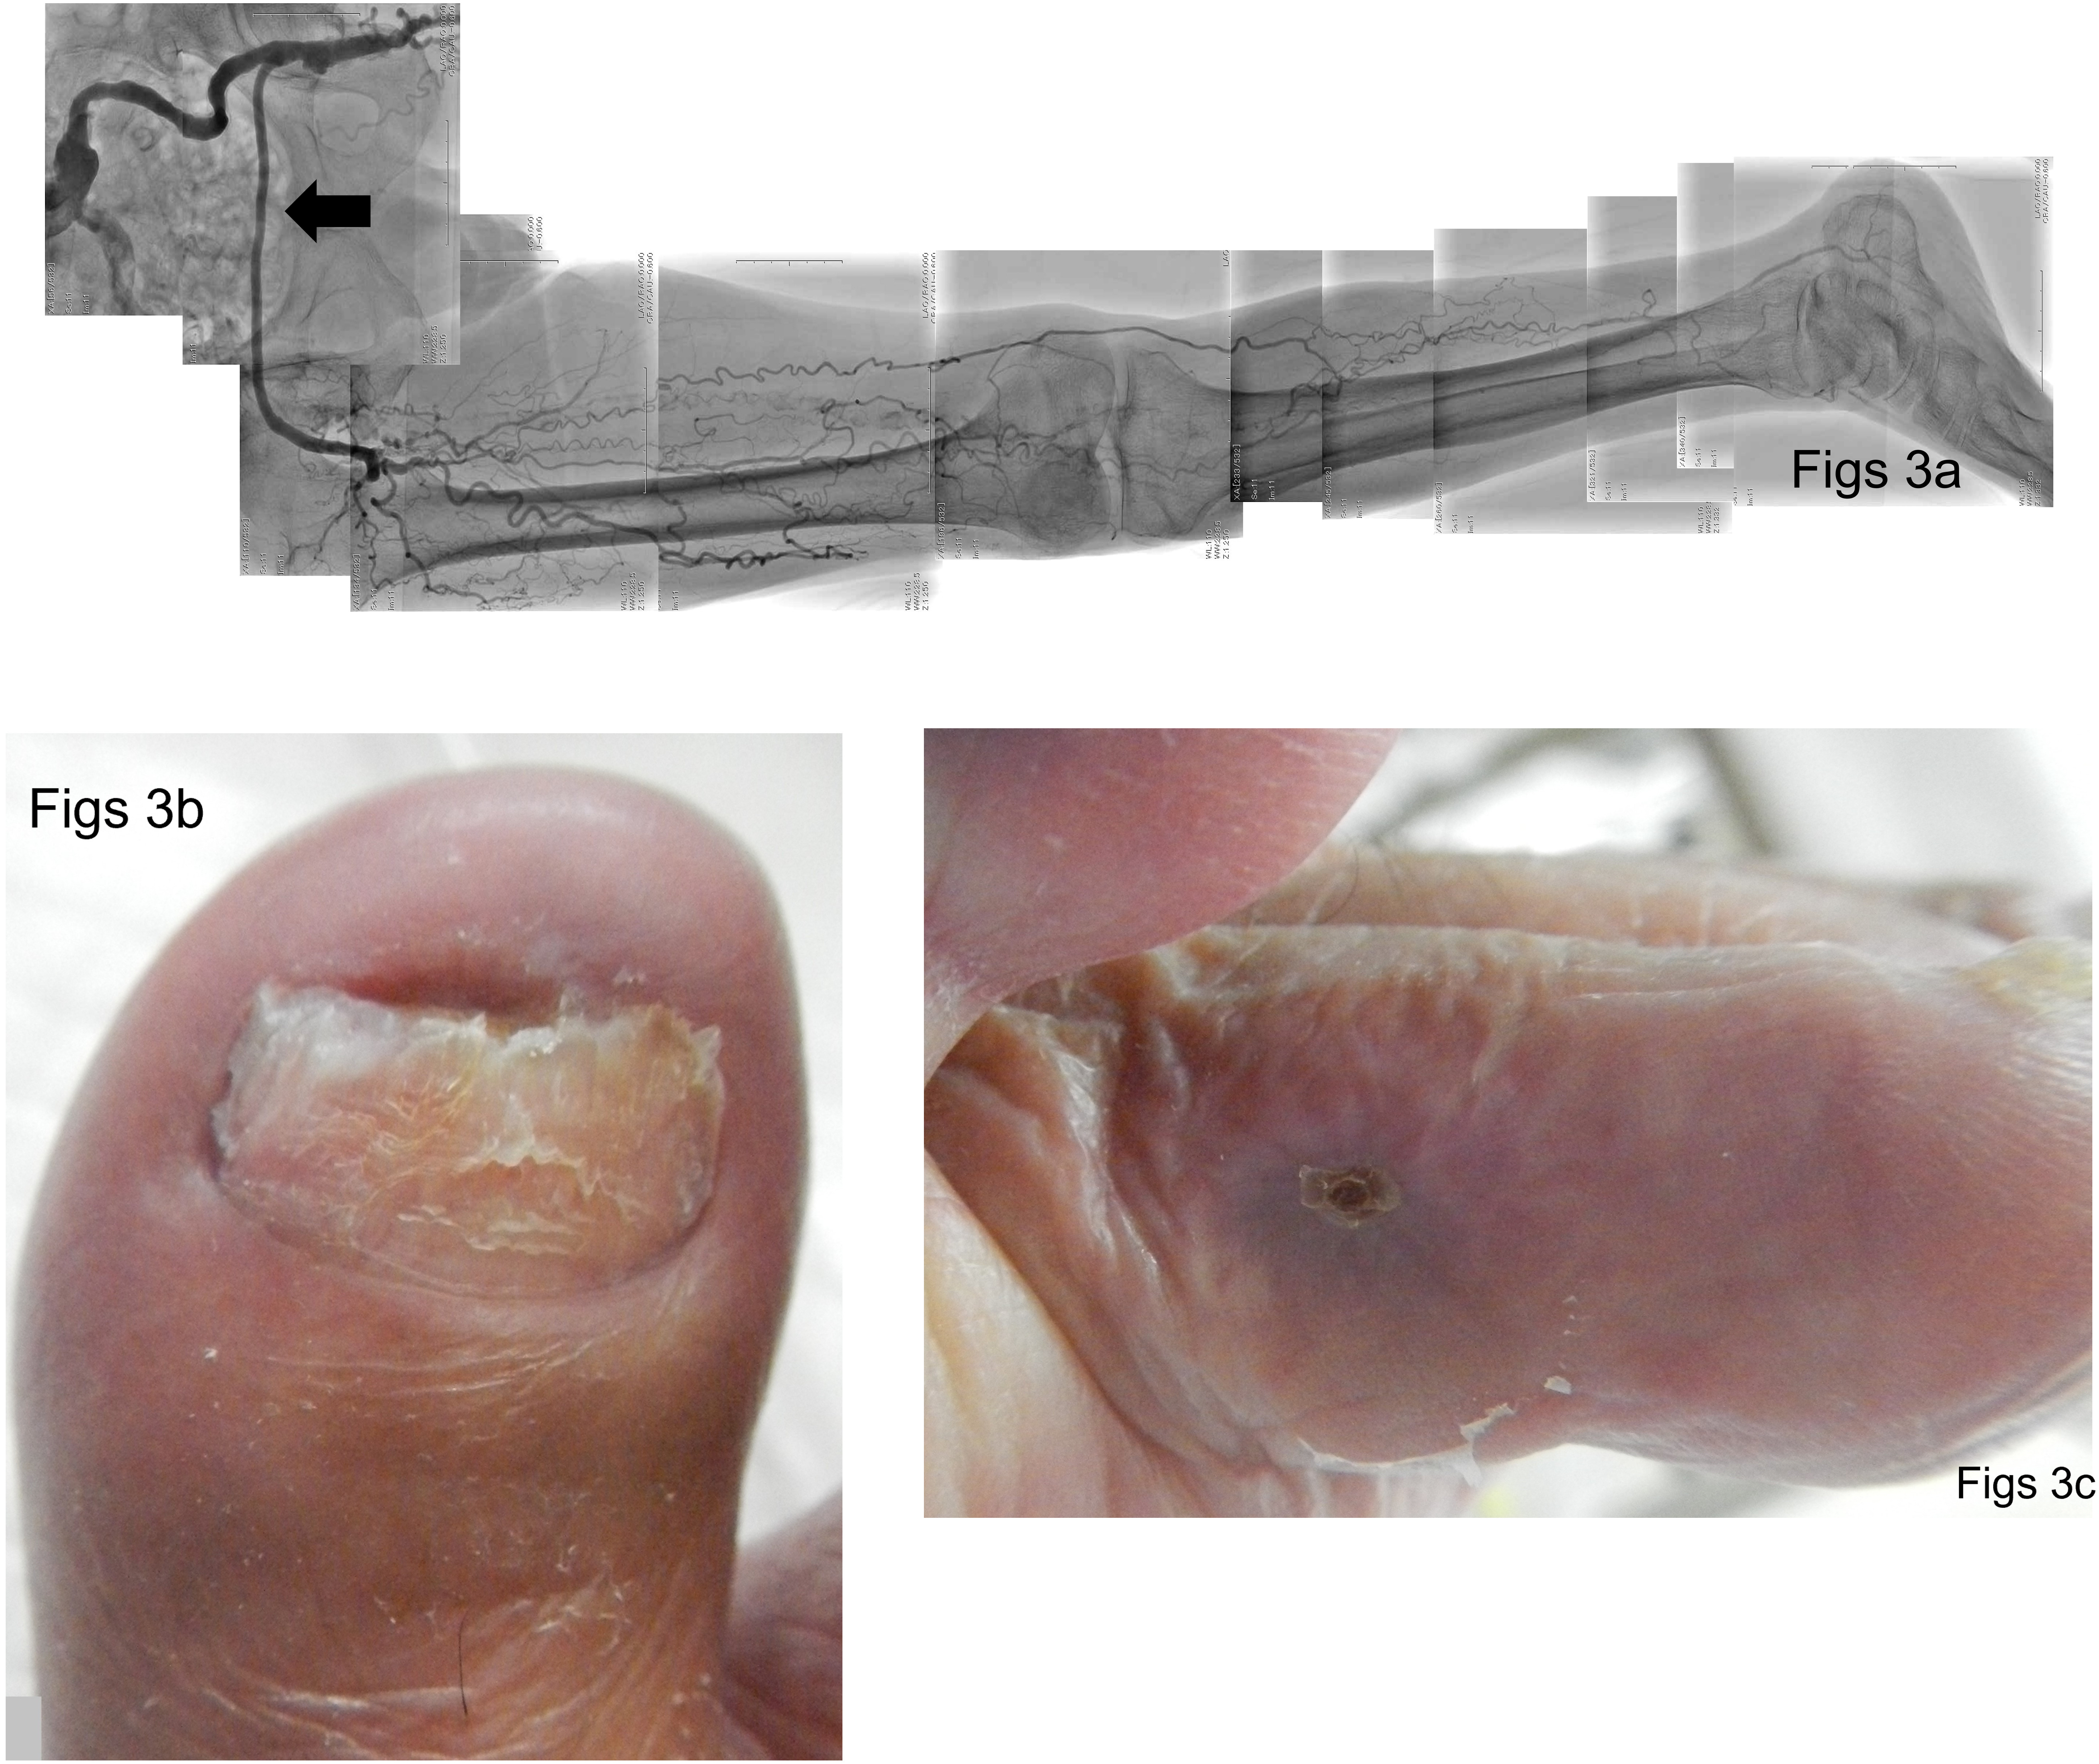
Fig. 3 Postoperative enhanced computed tomography and toes in 6 months. (a) The graft (➡) from the left common femoral artery to the right LFCA was patent. (b, c) The ulcers visually disappeared 6 months postsurgery.
